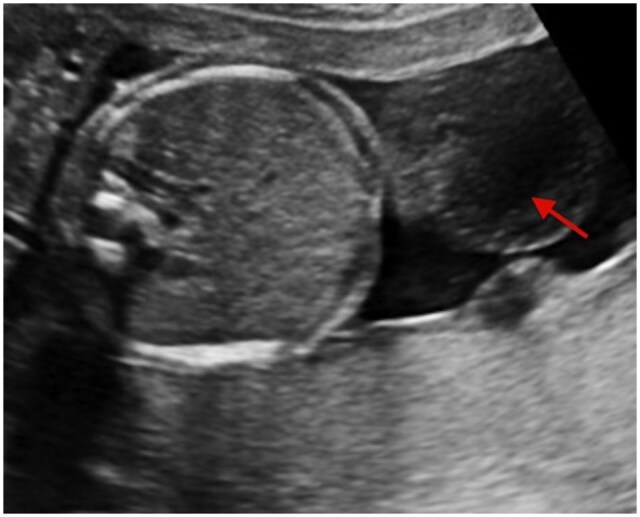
Antenatal ultrasound image demonstrating a Wharton’s jelly cyst (red arrow) within the umbilical cord in the same case of congenital hernia of the umbilical cord, seen as a well-defined anechoic cystic lesion without internal vascularity, distinct from the umbilical vessels.
